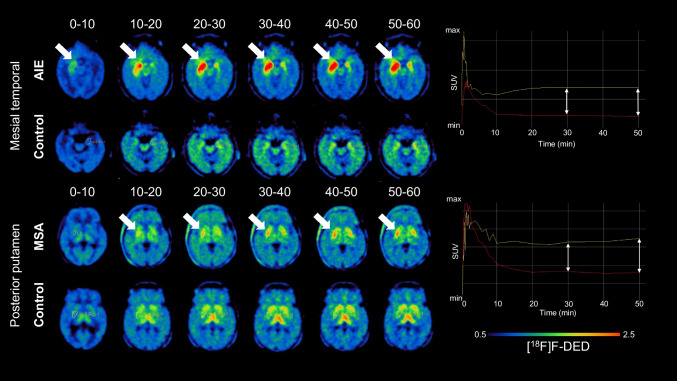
Trajectories of relative [^18^F]F-DED uptake during 60 min scan duration. Upper two rows show [^18^F]F-DED SUVr images in the axial plane of the mesial temporal lobe (MTL) for one patient with autoimmune encephalitis (AIE) in comparison to one control. Lower two rows show [^18^F]F-DED SUVr images in the axial plane of the posterior putamen (pPUT) for one patient with multiple system atrophy (MSA) in comparison to one control. Each image represents a single 10-min frame. Time-activity-curves on the right show SUV in target (yellow) and reference (red) regions as a function of time (50 min indicate the start of the last frame). PET-positive target lesions are indicated by the white arrows
